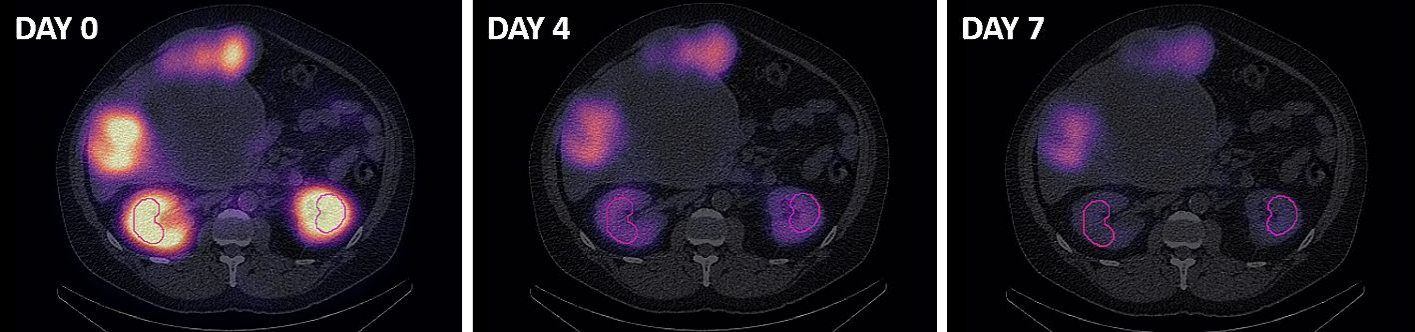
A small volume of interest drawn within the left and right kidneys on the day 0 SPECT and propagated onto the day 4 and 7 SPECT images
